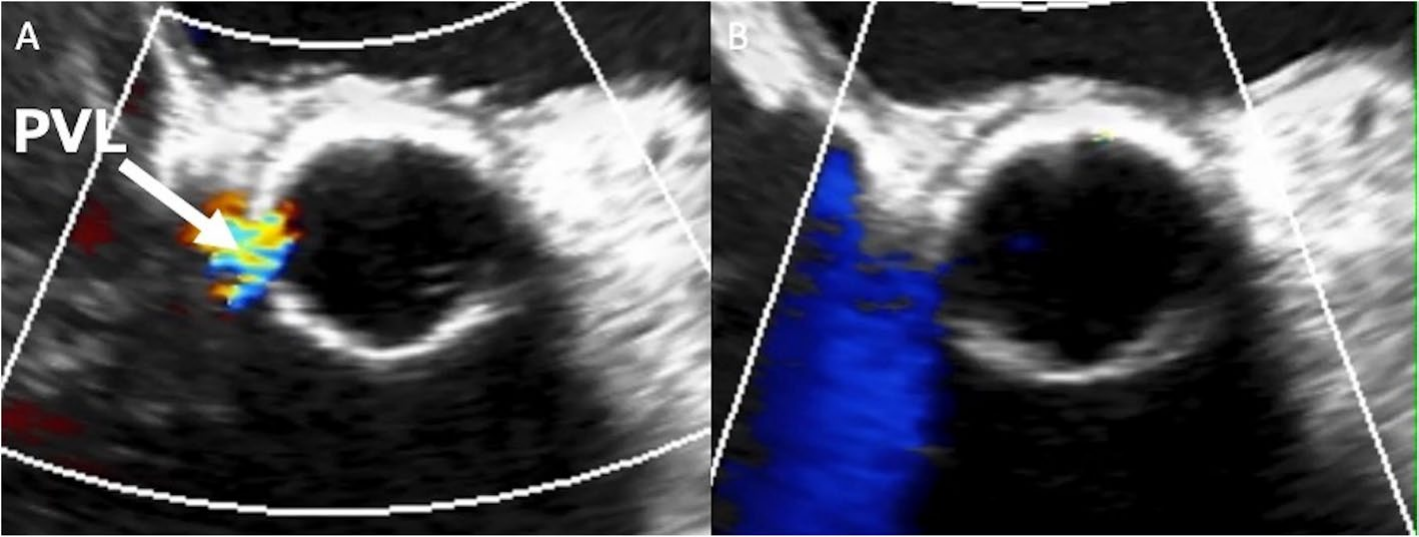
Intraoperative TEE in Case 2. In Case 2, intraoperative transesophageal echocardiography showed (A) a moderate paravalvular leak at the right coronary cusp side, and B the leakage resolved after re-ballooning the sealing frame
